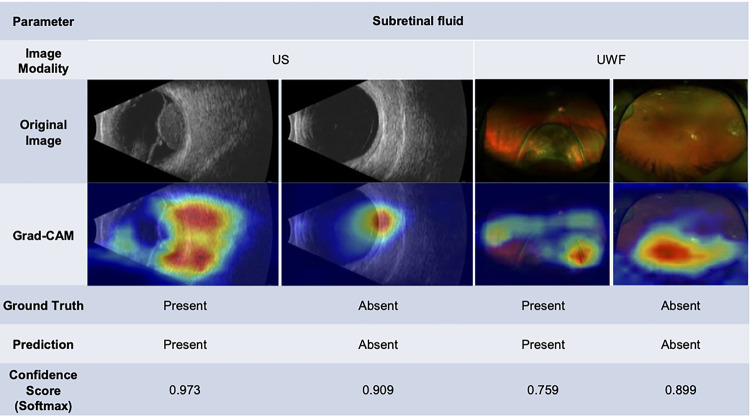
Ultrasound (US) and ultra-widefield fundus (UWF) original images, with a Grad-CAM saliency map showing the areas of highest probability for the subretinal fluid prediction model.
